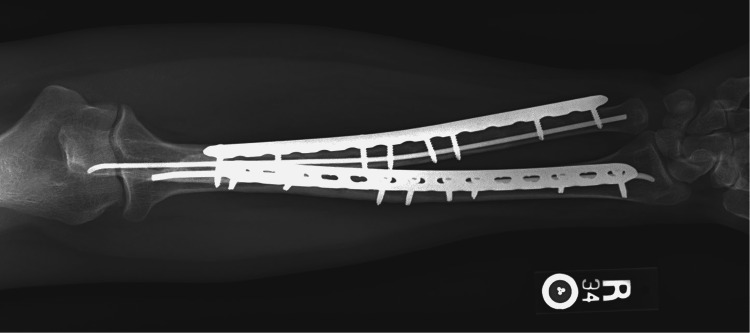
Anteroposterior forearm radiograph at 18 months after definitive surgery.
